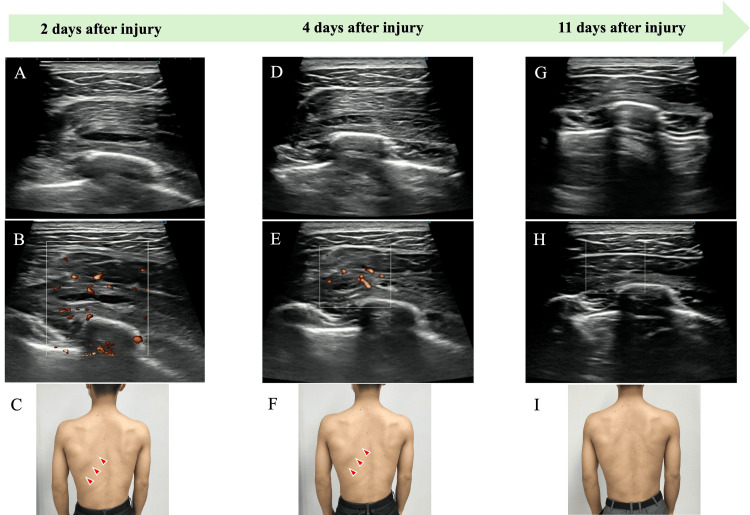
Serial ultrasonographic and clinical observations On days 2-4 post-injury, a hypoechoic area, increased blood flow signals, and localized swelling were observed. By day 11, the hypoechoic area and blood flow signals had resolved, and swelling had markedly decreased. (A) Ultrasound image two days after injury. (B) Color Doppler image two days after injury. (C) Clinical appearance two days after injury. (D) Ultrasound image four days after injury. (E) Color Doppler image four days after injury. (F) Clinical appearance four days after injury. (G) Ultrasound image 11 days after injury. (H) Color Doppler image 11 days after injury. (I) Clinical appearance 11 days after injury.
